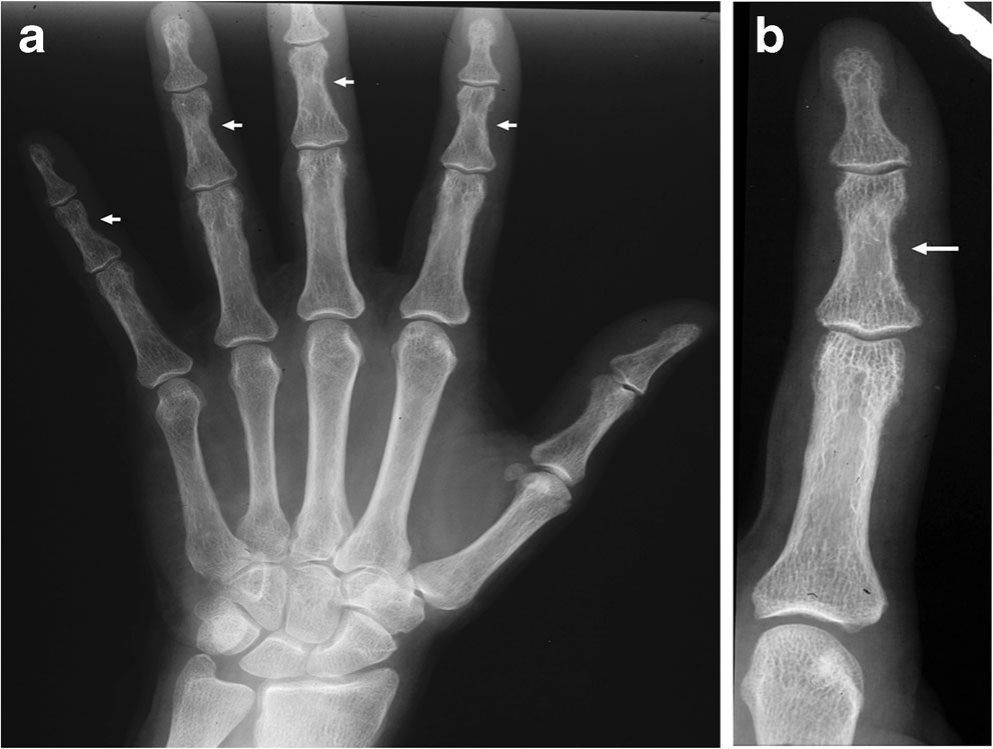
a, b: a Radiographic image of the left hand in a patient undergoing chronic haemodialysis. b Magnification. Secondary hyperparathyroidism with bone resorption along the radial aspects of the middle phalanges of the fingers (*arrows*)
